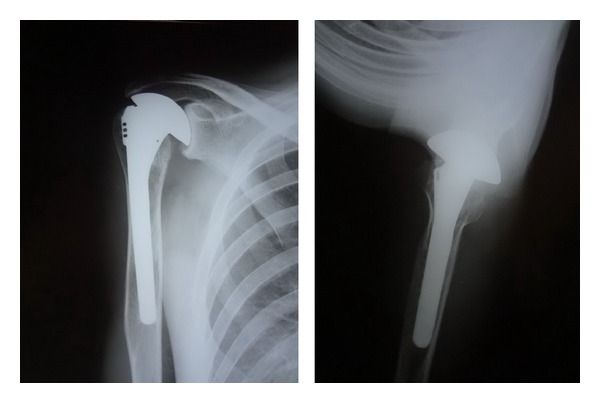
The follow-up radiographs at 60 months postoperatively.
